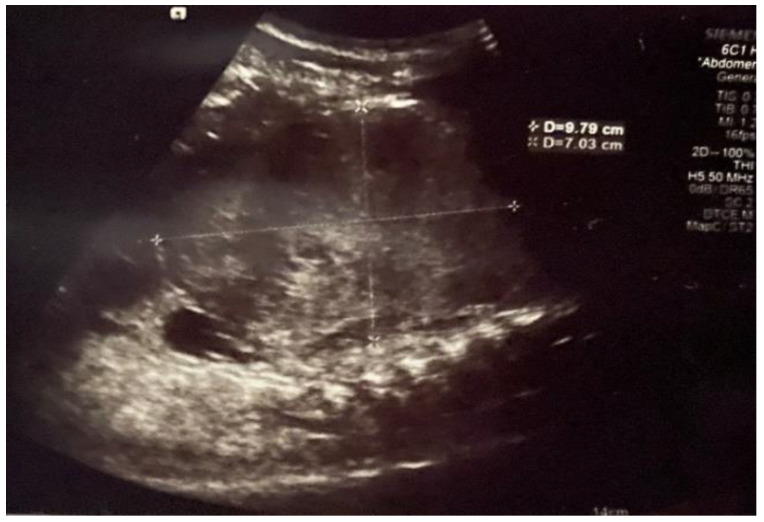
Initial abdominal ultrasound showing a large heterogeneous liver mass.
